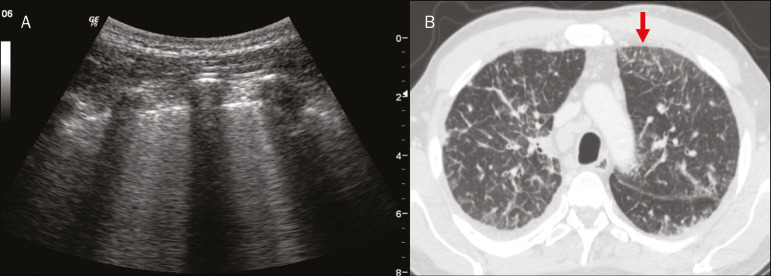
A: Ultrasound image shows coalescent B-lines (transducer in the left hemithorax, at the site of the arrow in B). B: Chest CT scan of the same patient shows thickening of the interlobular and intralobular septa due to carcinomatous lymphangitis, and some ground-glass opacities.
